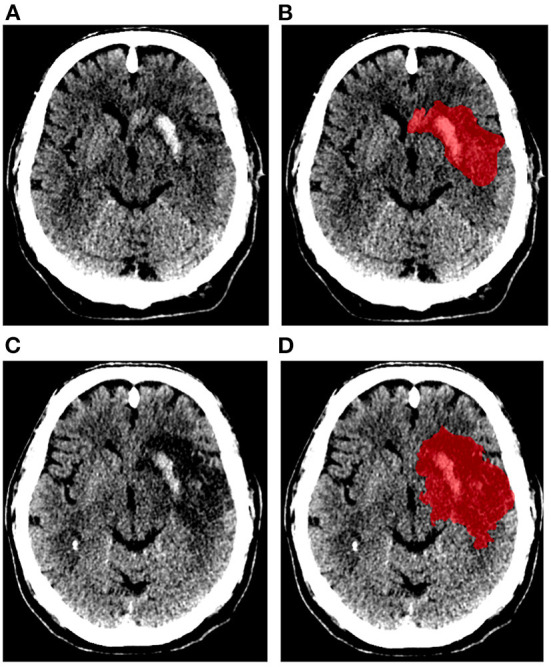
Example of ischemic lesion segmentation (represented in red). Non-contrast CT scans and segmentation of ischemic lesion obtained after 24 h (A,B), and 1 week (C,D) of stroke onset.
